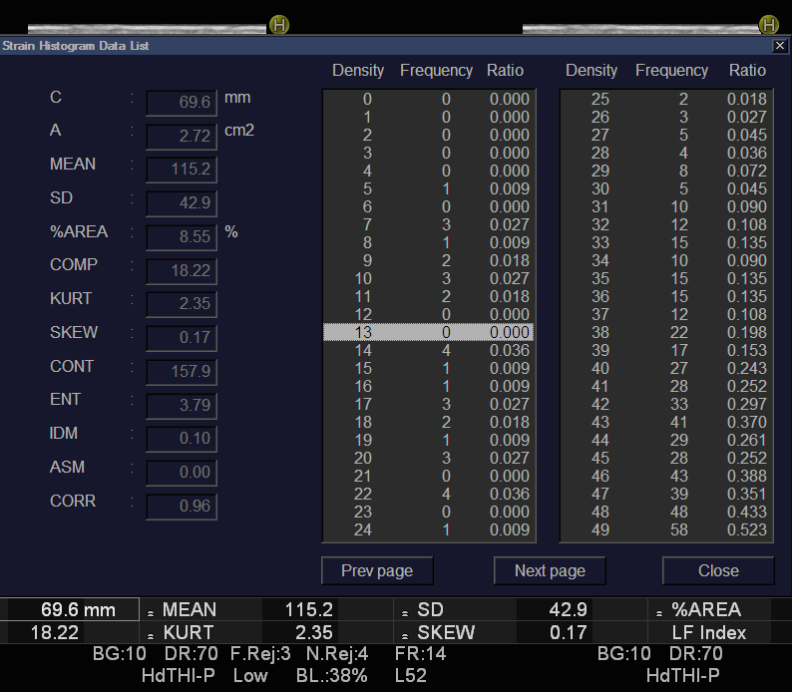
Elastic parameters calculated by an ultrasound system in order to characterize liver stiffness
